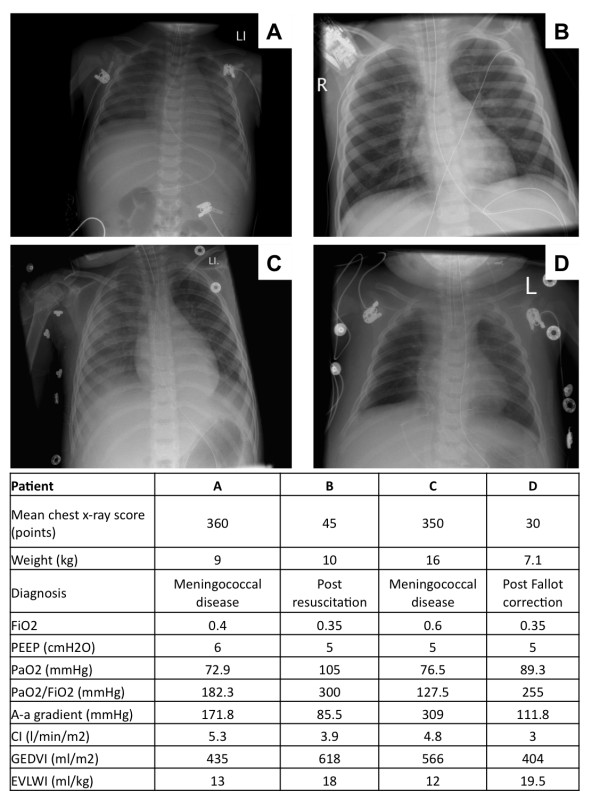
Example of chest x-rays and related variables in four children with the lowest and highest chest x-ray score.
